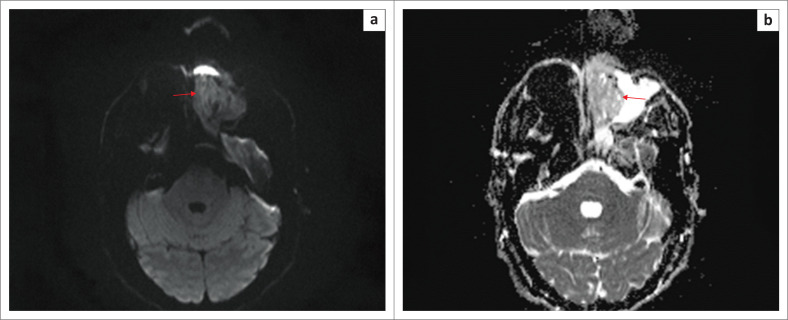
(a) Diffusion-weighted imaging B-1000/mm. The mass shows restricted diffusion (red arrow). There is an area of susceptibility artefact anteriorly and at the posterior skull base. (b) Axial ADC map MRI image shows a lobulated left nasal mass with restricted diffusion and small foci of T2 shine-through, ascribed to small foci of intratumoural cysts or necrosis (red arrow).
